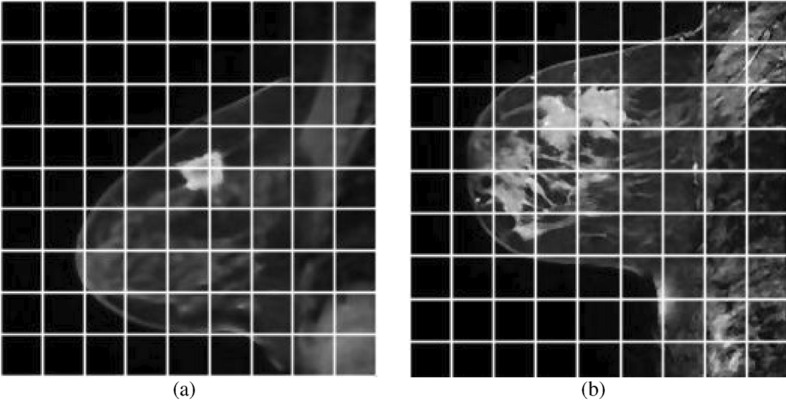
De-noised images of original T2 weighted sagittal DCE-MRI in Fig. 5.
